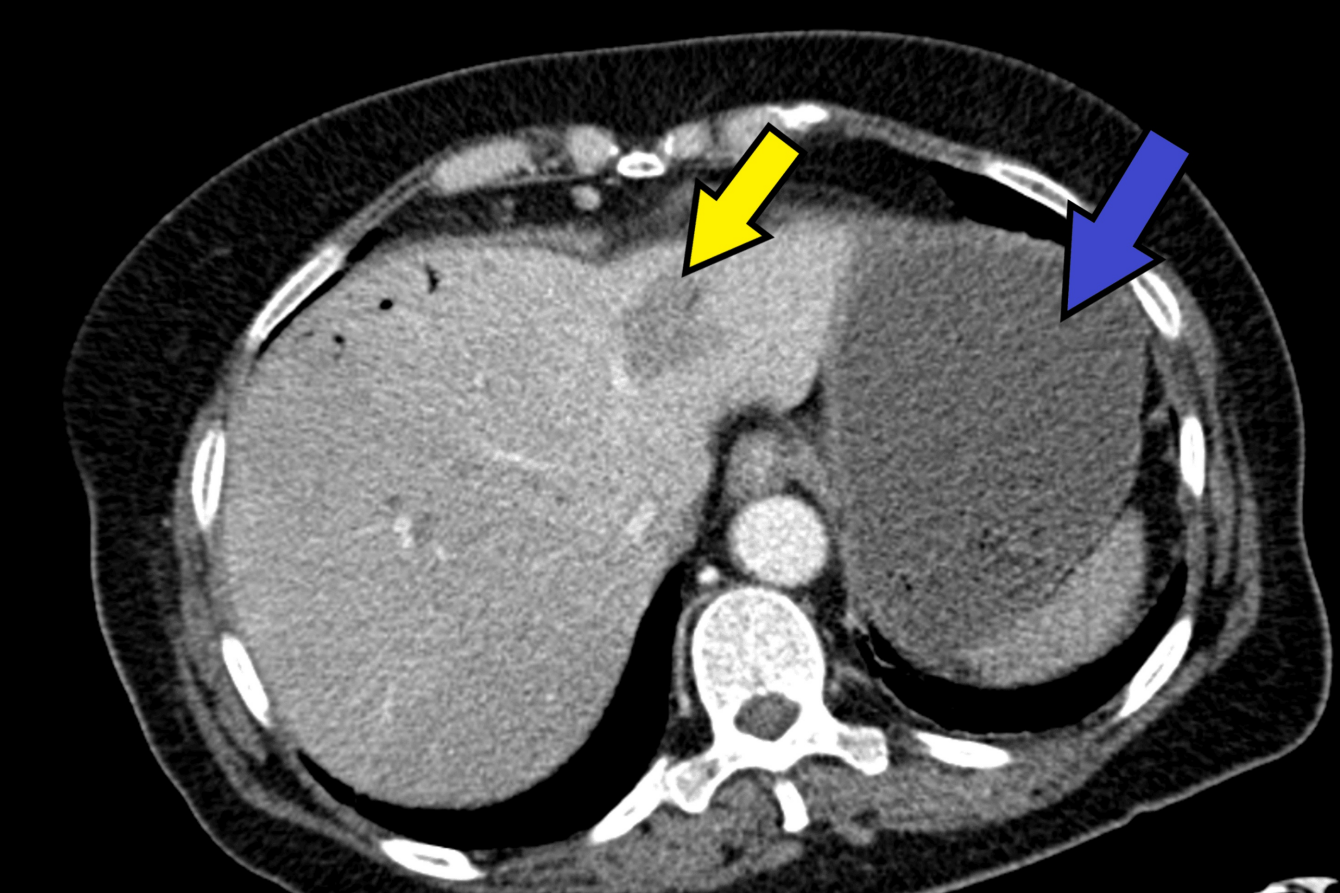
Contrast-enhanced abdominopelvic CT scan Blue arrow: Splenic infarcts; Yellow arrow: Presence of portal venous gas involving segments IV and VIII of the liver
